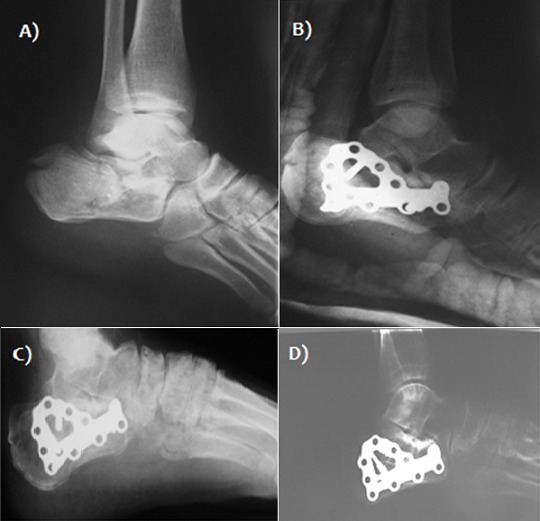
A) radiographie de cheville (profil) montrant une fracture du calcanéum stade IV; B) radiographie post opératoire-ostéosynthèse par une plaque anatomique en Y; C) contrôle radiologique de la fracture à 3 mois; D) contrôle radiologique de la fracture à un an
